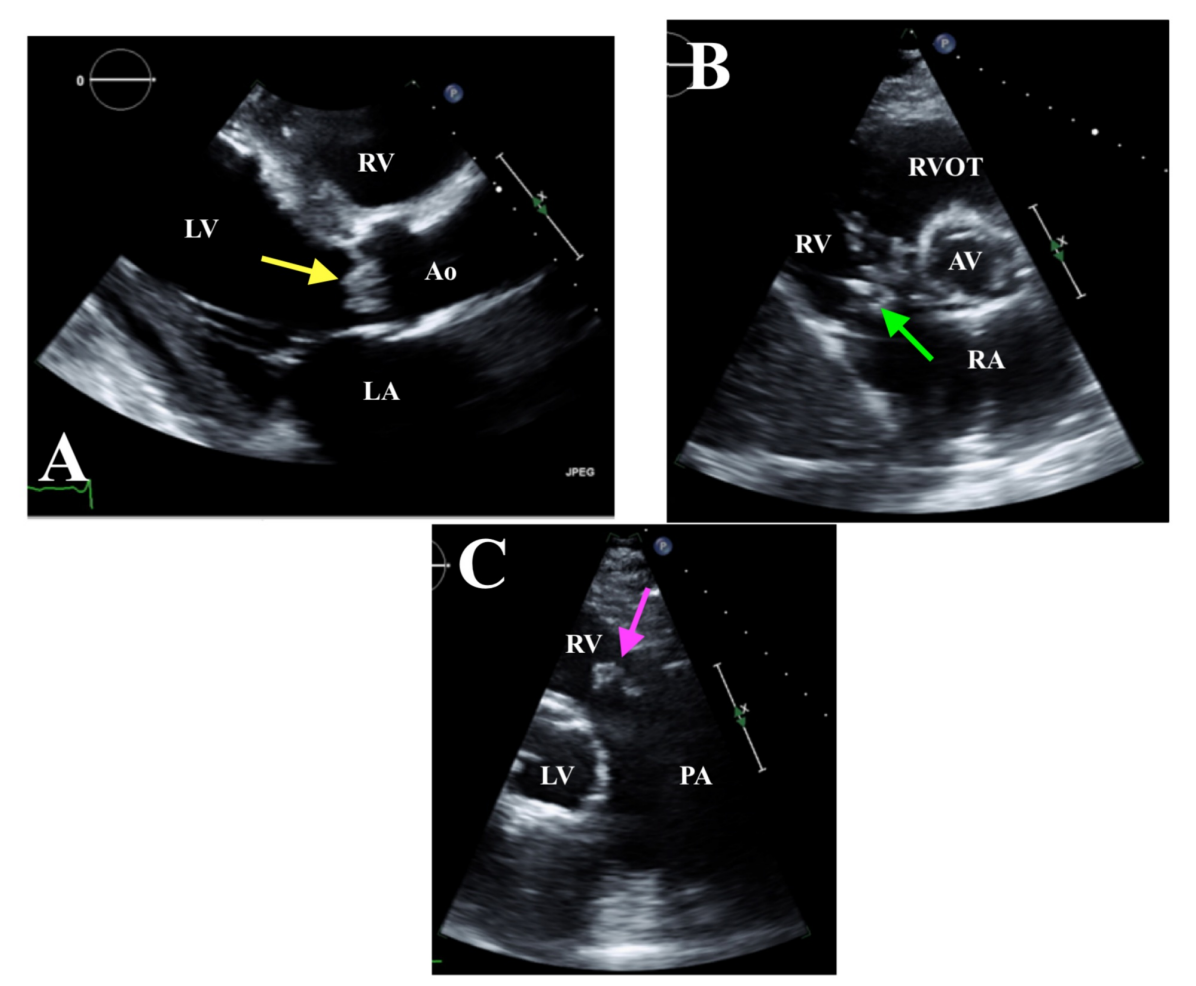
TTE. A: Parasternal long axis view with AV vegetation measuring 0.9 cm x 0.8 cm (yellow arrow). B: Parasternal short axis view showing tricuspid valve vegetation measuring 1.4 cm (green arrow). C: RVOT view with pulmonic valve vegetation measuring 1.4 cm x 1.1 cm (pink arrow). TTE, transthoracic echocardiogram; LA, left atrium; LV, left ventricle; Ao, aorta; AV, aortic valve; RV, right ventricle; PA, pulmonary artery; RVOT, right ventricular outflow tract
